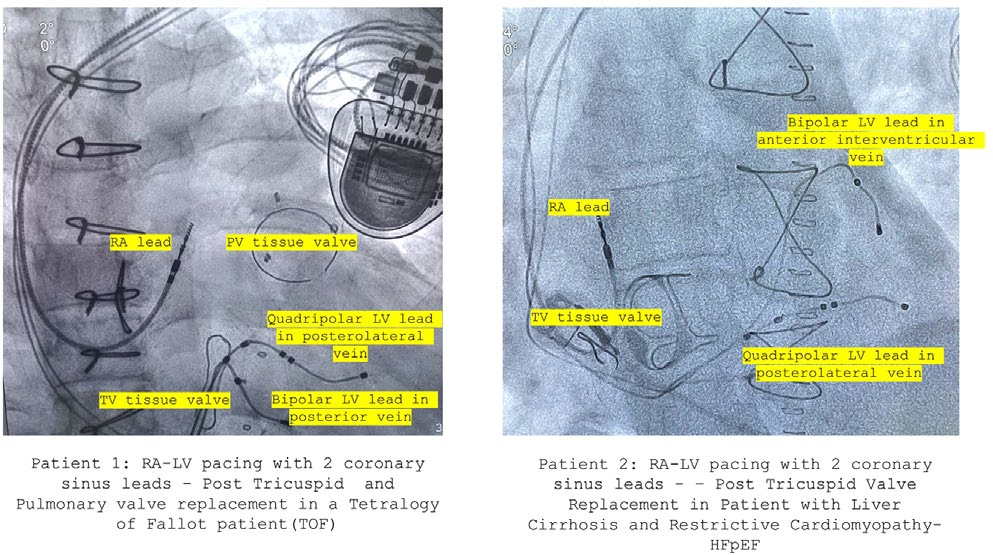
## EXPLORING PREDICTIVE FACTORS FOR SURVIVAL OUTCOME IN CARDIAC ARREST PATIENTS UNDERGOING EXTRACORPOREAL MEMBRANE OXYGENATION: A RETROSPECTIVE ANALYSIS
